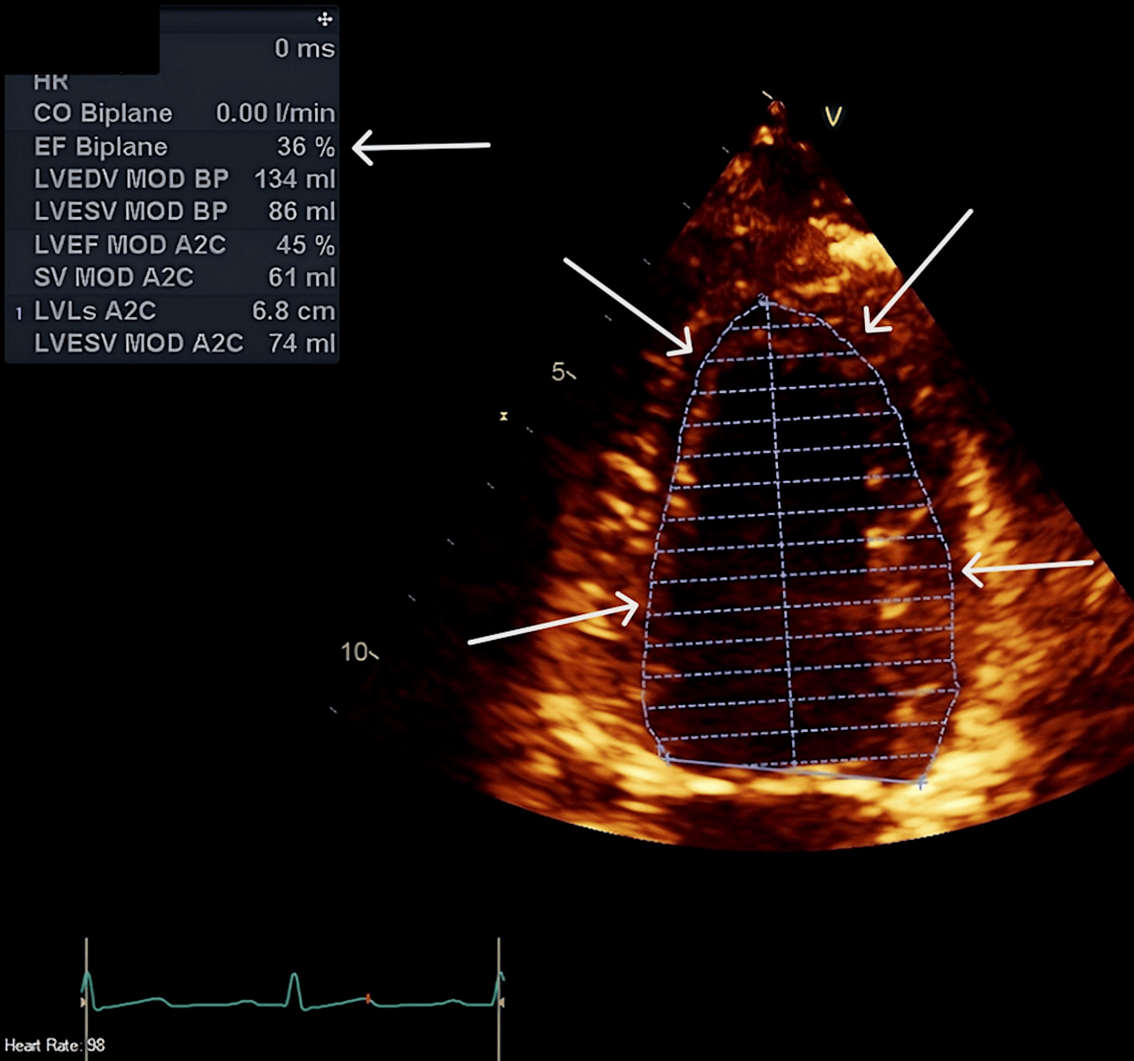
2D TTE Day 0 showing normal left ventricular cavity size with severe LV systolic dysfunction by Simpson's biplane LVEF 36%. Arrows delineating the left ventricular internal borders used to calculate the ejection fraction of 36% using Simpson's biplane. EF: ejection fraction by Simpson's biplane method; TTE: transthoracic echo
